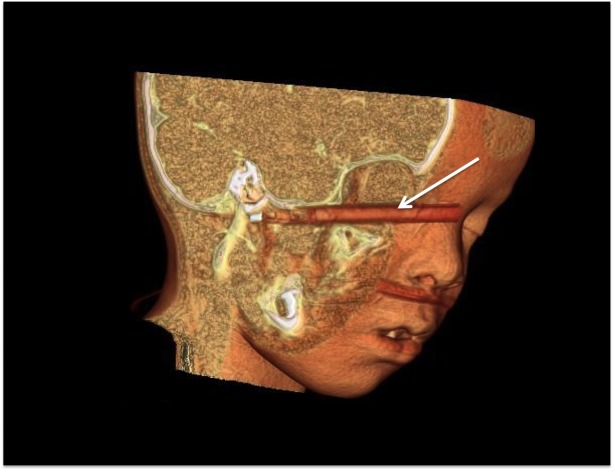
3D CT reconstruction of a child who fell on a writing pen. The pen entered the right orbit, then followed the skull base to end just anterior to the internal carotid artery. 3D CT: three-dimensional computed tomography
